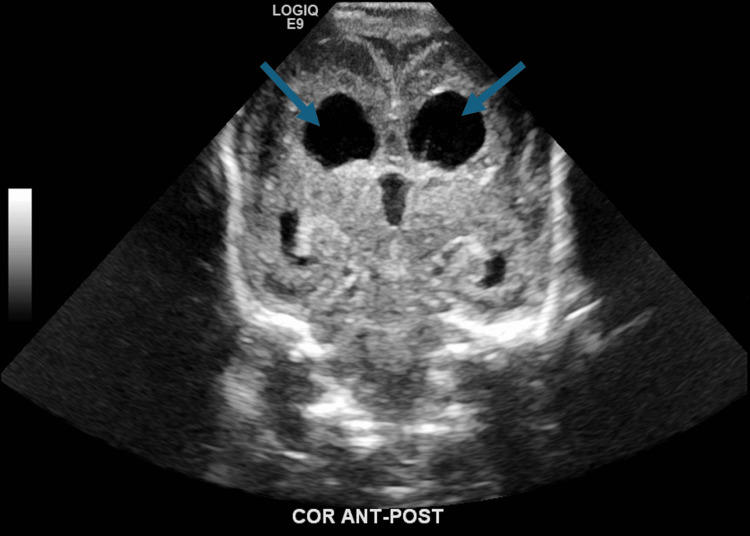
Still image from the head ultrasound on day of life seven with evident congenital abnormality of the midline structures noted with secondary prominence of the ventricles (blue arrows)
